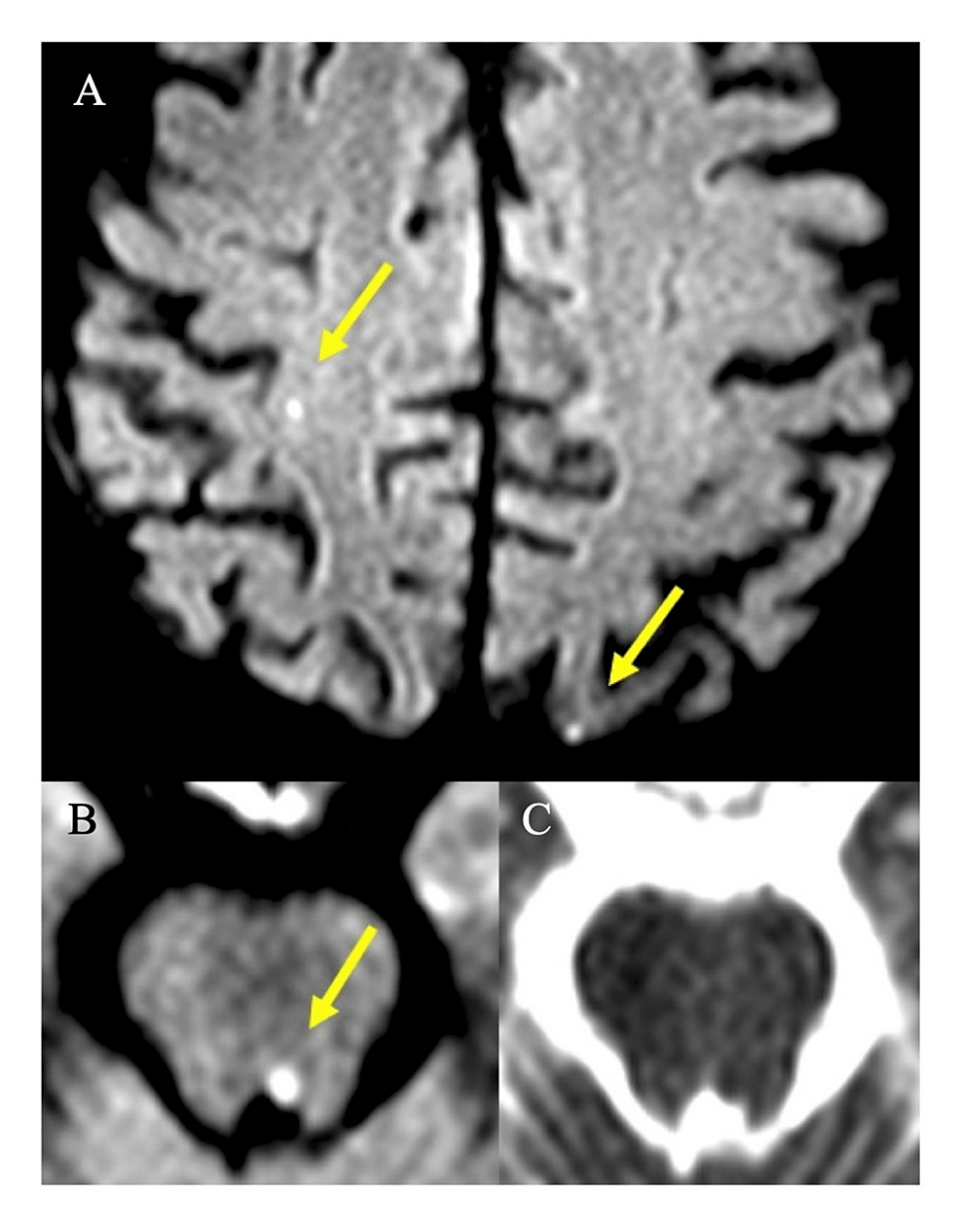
Cerebral microemboli on MRI following TAVI Diffusion-weighted imaging at b1000 showing small infarcts (arrows) in the right parietal left occipital lobes (Figure 2A) and left medial longitudinal fasciculus (Figure 2B). Apparent diffusion coefficient of the left medial longitudinal fasciculus stroke (Figure 2C). TAVI: transcatheter aortic valve implantation
